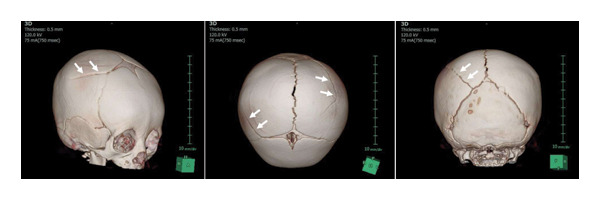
Head three‐dimensional computed tomography clearly shows linear biparietal skull fractures (white arrows).
